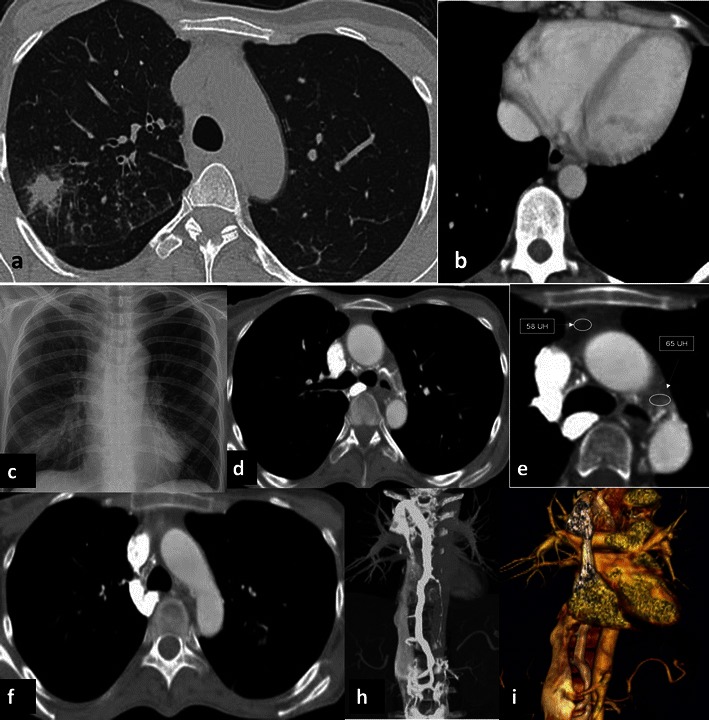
Atypical mycobacterial infection: induced fibrosis mediastinitis. A 22-year-old female, affected by celiac disease, underwent CT for fever and cough. It showed a consolidation and tree-in-bud pattern in the right upper lobe (a). The heart and azygos vein were normal (arrow) in size (b) . Bronchoscopy identified an atypical mycobacterial infection. One year later, she underwent a chest X-ray, which showed an enlargement of the superior mediastinum even though the heart size was normal (c). Contrast-enhanced CT scan showed a significant enlargement of the azygos vein, not present in the prior exam (d–f), associated with an increased density of the mediastinal tissue (e). A retrograde, early opacification of the azygous vein can be seen in the axial (d–f) and coronal MPR (h) and VR (f). In the coronal view, the inferior vena cava was enlarged as well. The patient had a fibrosing mediastinitis caused by an atypical mycobacterial infection that induced a reduced calibre of the superior vena cava in the infra-azygos region and retrograde flow in the azygos system
